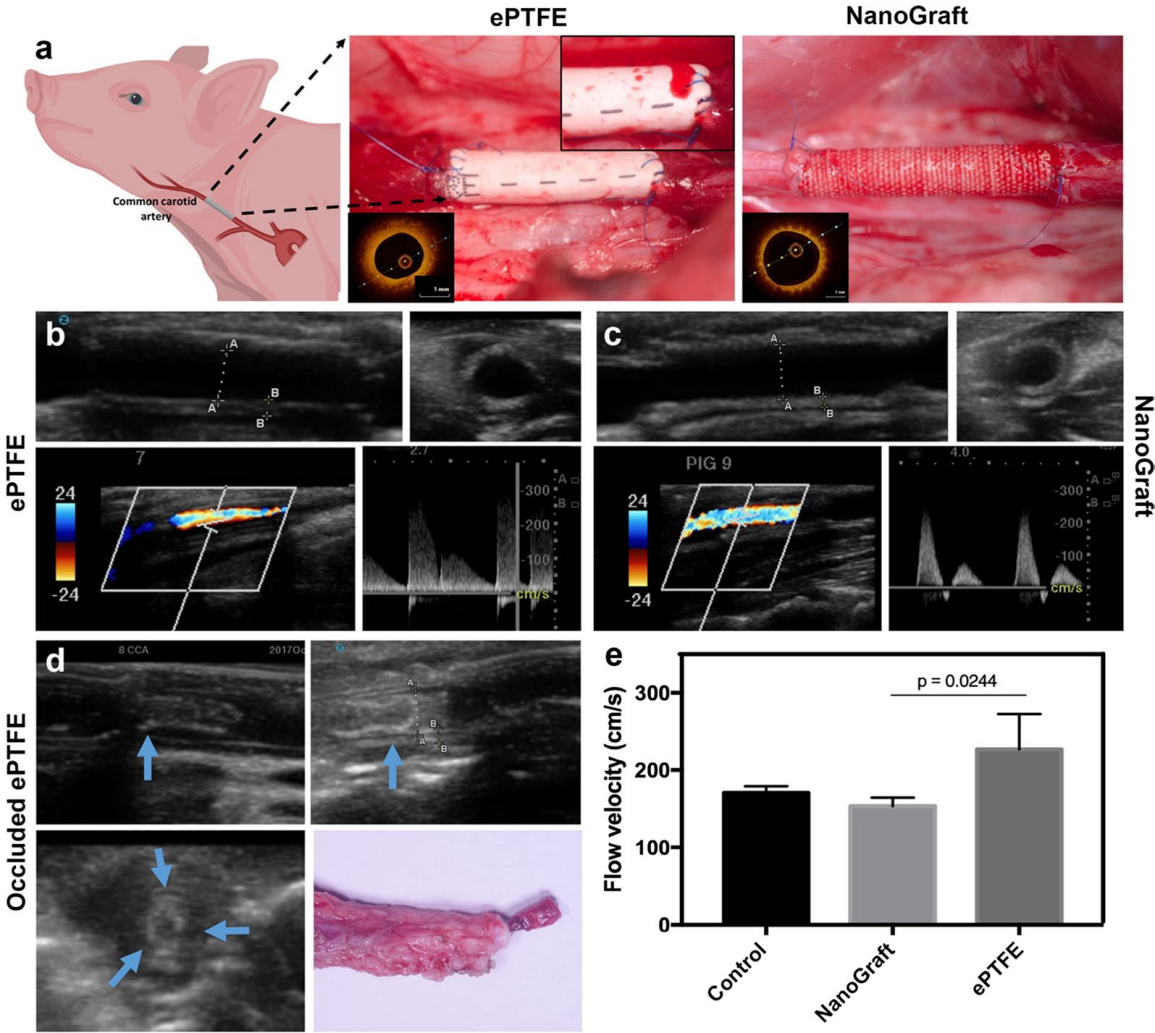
In-vivo implantation and imaging assessment of ePTFE and NanoGraft grafts: a direct representative picture of the ePTFE graft with oozing from suture line and NanoGraft with no oozing. Inset shows the OCT image of the patent grafts. Representative 2D Doppler of the longitudinal and cross-sectional view of b ePTFE and c NanoGrafts (distance A- 3.8 mm, B-0.7 mm), with the corresponding qualitative and quantitative evaluation of blood flow through the grafts (bottom in b and c). d 2D Doppler of the longitudinal and cross-sectional view of the occluded ePTFE grafts (blue arrows depict the regions with adherent clots) and its corresponding optical image. e Quantitative evaluation of blood flow through the patent synthetic grafts before and after implantation
